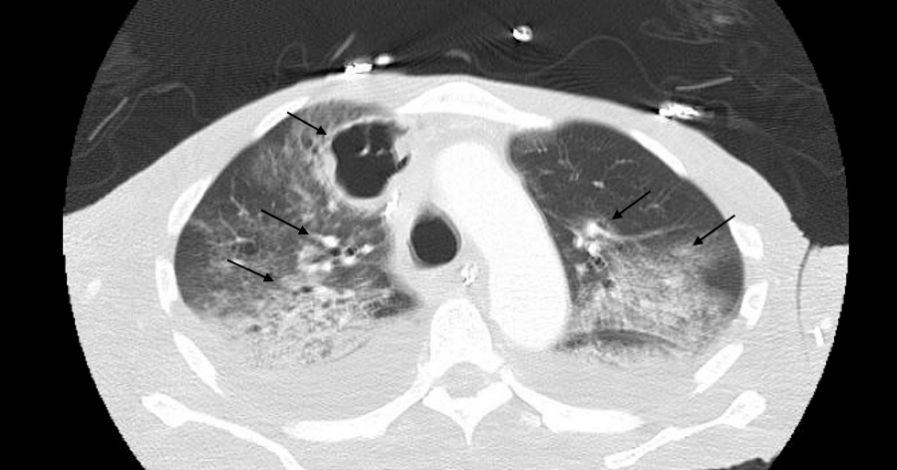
CT chest showing diffuse ground glass opacities
